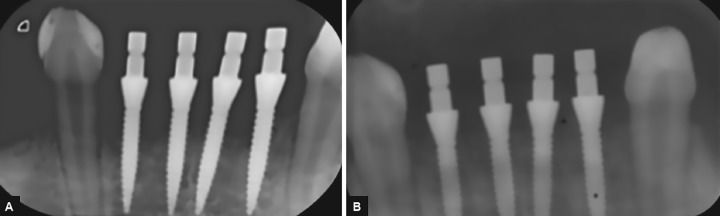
Two radiographic images taken for the same sector and at the same time. Note the change in the bone level due to modification of the contrast level
